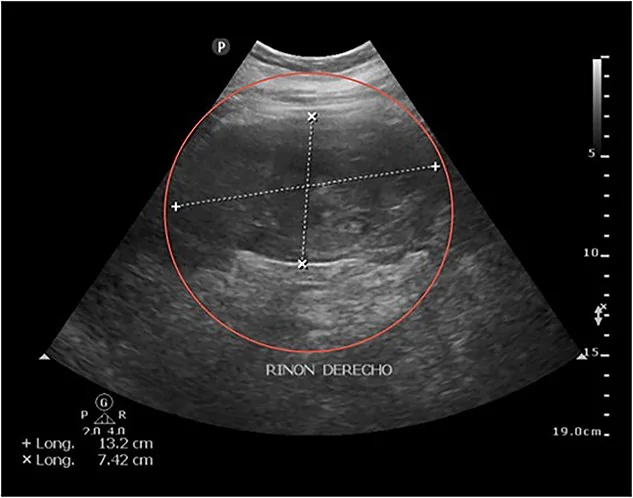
Ultrasonography. Right kidney compressed by a heterogeneous mass (circle). *Source: Image obtained by the authors.*
